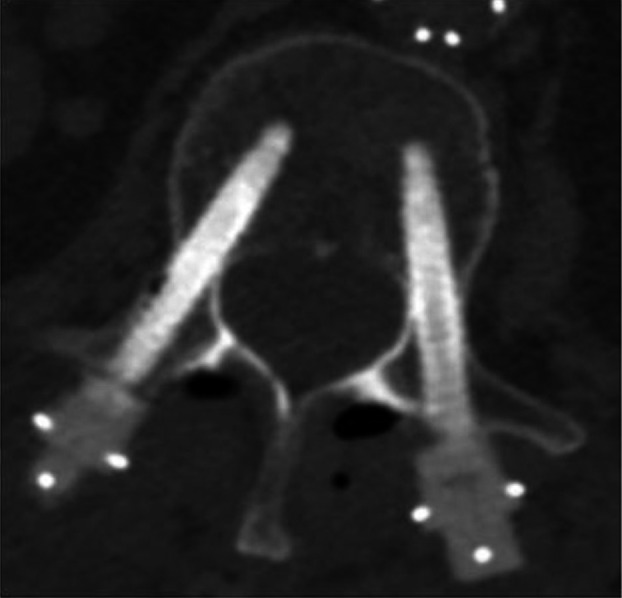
CT image of pedicle screws with titanium coating inserted into vertebra.
